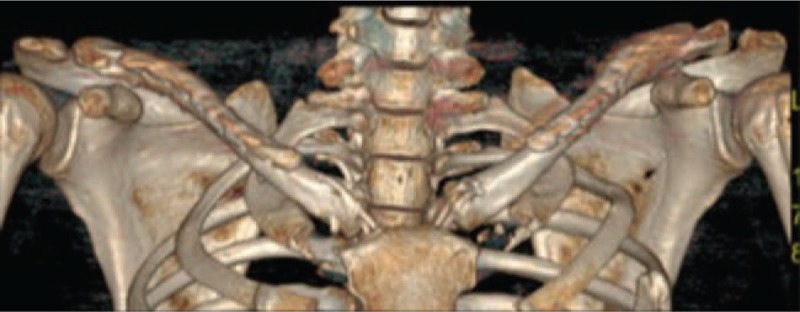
Three-dimensional computed tomography (CT) scans confirmed bilateral sternoclavicular joint (SCJ) reduction and alignment after operation 1 week. CT = computed tomography, SCJ = sternoclavicular joint.
